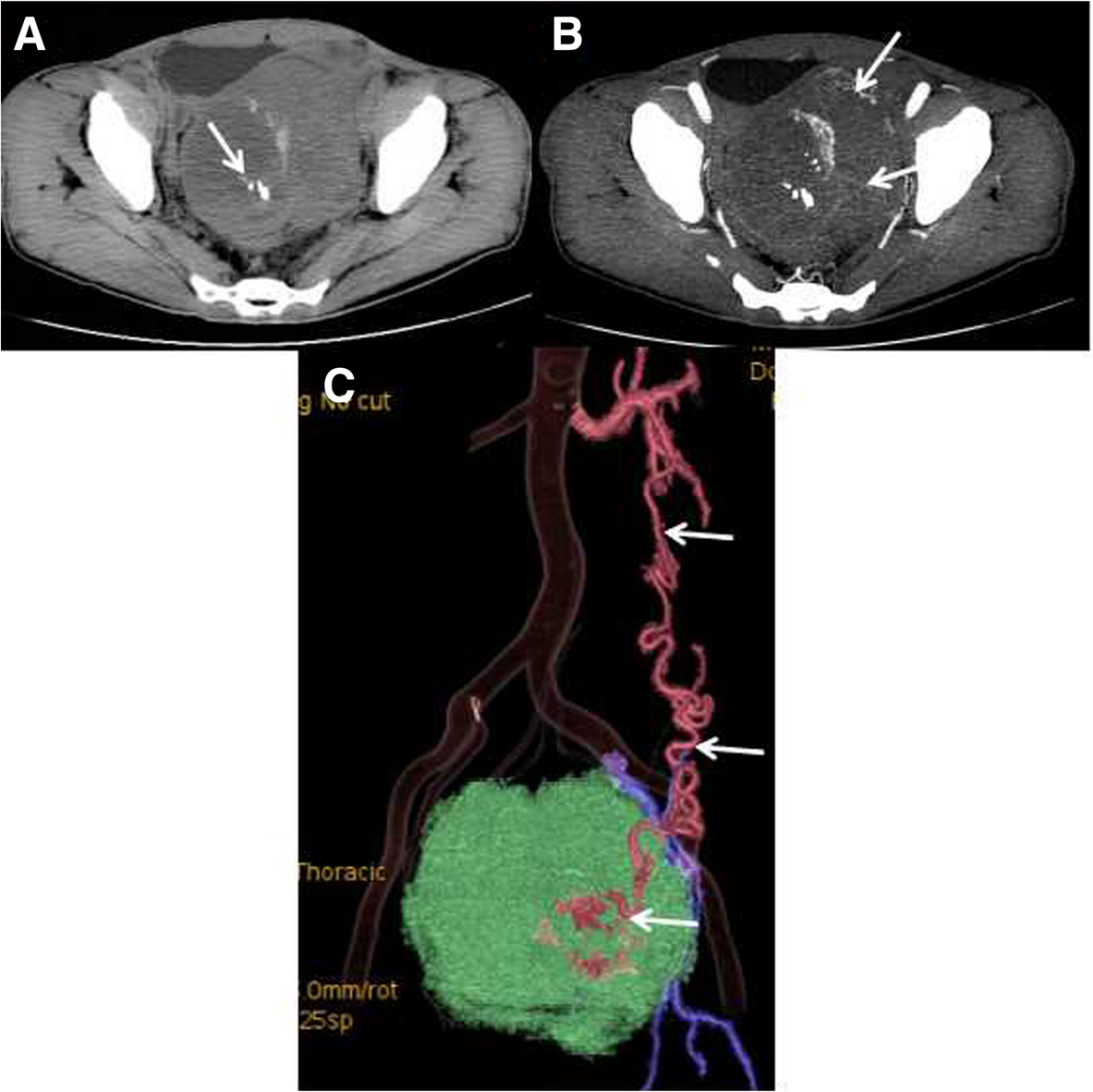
CT appearance of a pelvic undescended testis secondary seminoma. a A CT plain scan showed a huge and round soft tissue mass in the pelvis, with a clear margin and inhomogeneous density. Small dots or striped calcifications and irregular low-density areas were visible in the tumor. b In the arterial phase, vascular penetration was visible in the lesion (arrow). The solid part of the tumor was slightly enhanced. c SSD reconstruction showed that a thickened and tortuous left testicular artery was the supply artery of the tumor. The testicular arteries were clearly displayed, whereas the testicular veins were poorly displayed, which was consistent with the “testicular vascular pedicle sign”. “Testicular vascular pedicle sign” means testicular arteries were clearly displayed, whereas the testicular veins were poorly displayed, and the accessory blood vessels was relatively normal developed and displayed in contrast or enhanced CT
